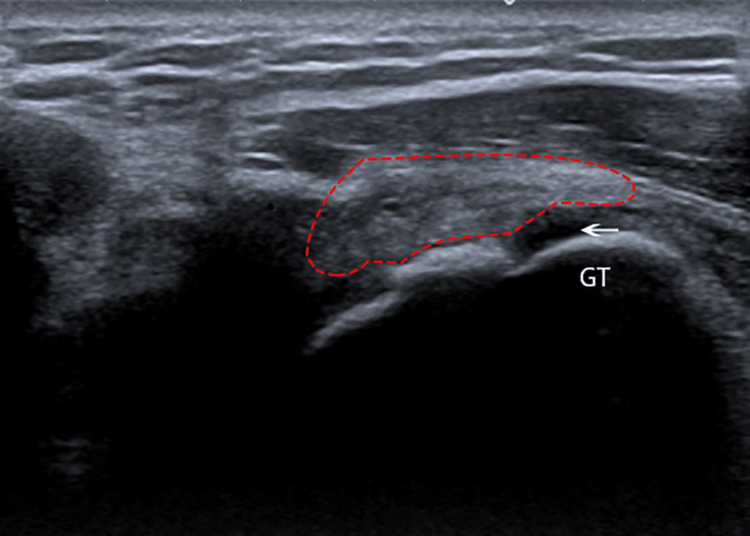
Ultrasound of the shoulder revealing a partial tear of the supraspinatus tendon from the greater tubercle of the humeral head. GT: Greater tubercle of humerus; Red outline: Supraspinatus tendon; White arrow: Partial tear of supraspinatus tendon from its attachment
